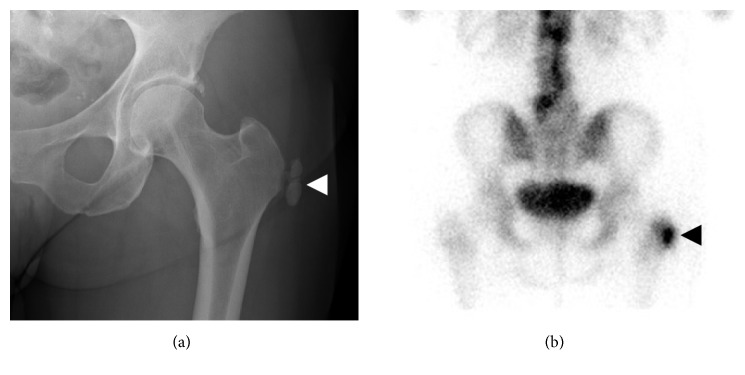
Appearance of calcific bursitis on technetium-99m bone scan. (a) A large conglomeration of soft tissue calcifications reside in the region of the left greater trochanter bursa on AP hip radiograph (white arrowhead). (b) The calcifications demonstrate exuberant increased radiotracer uptake on the delayed phase of technetium-99m MDP bone scan (black arrowhead).
